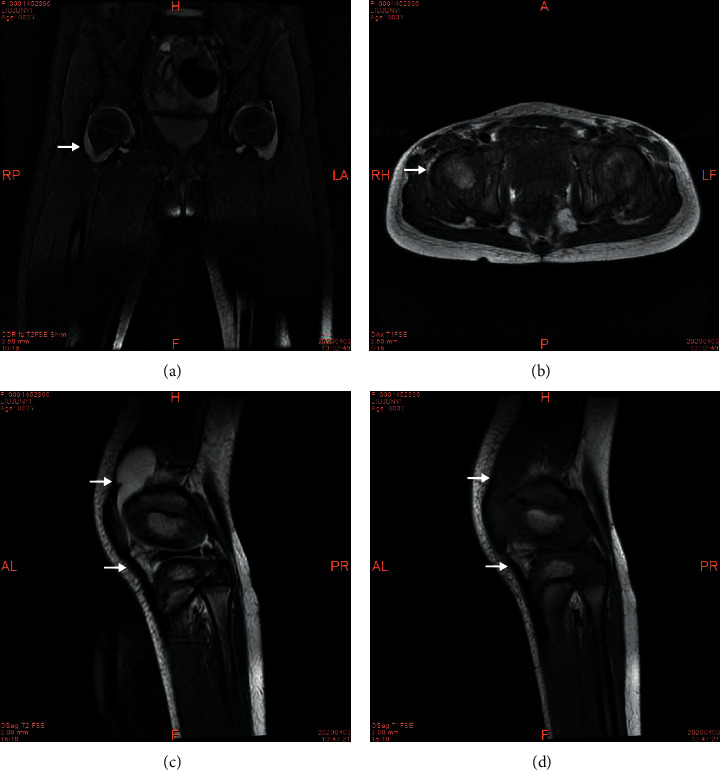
T1-weighed, T2-weighed, and Fat-suppressed T2-weighted imaging in the acute phase of Kawasaki disease. There were a long T1 signal and long T2 signal in the bilateral hips (a), (b), knees, and bursae suprapatellaris (c), (d), which indicated arthritis with effusion.
